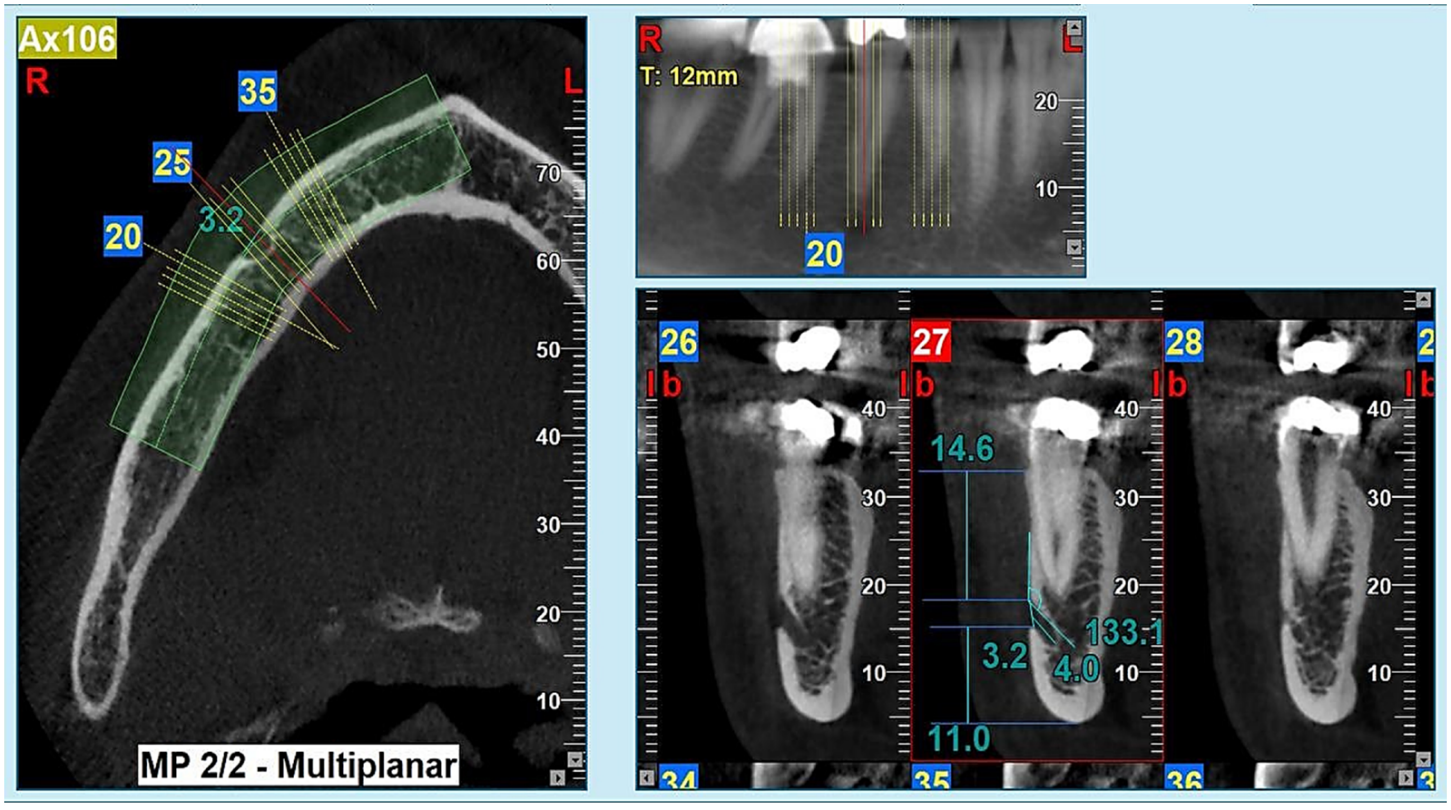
Assessment of mental foramen and canal using cone-beam computed tomography imaging technique.
